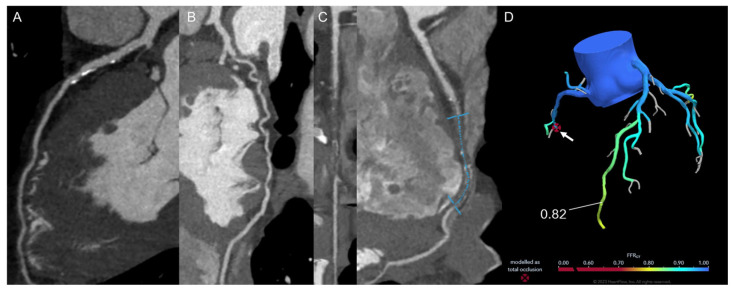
A 60-year-old man with family history of CAD, hypertension and dyslipidemia complained of recent onset of effort dyspnea. CCT showed non-obstructive disease of the left anterior descending artery (A) and left circumflex artery (B), and a long (50 mm) chronic total occlusion of mid-right coronary artery (C) with collateral supply from the left circumflex artery. FFR-CT showed absent functional relevance of the other stenosis and correctly displayed the occlusion (D). The patient underwent percutaneous recanalization of the right coronary artery with multiple drug-eluting stents implantation with symptom resolution.
